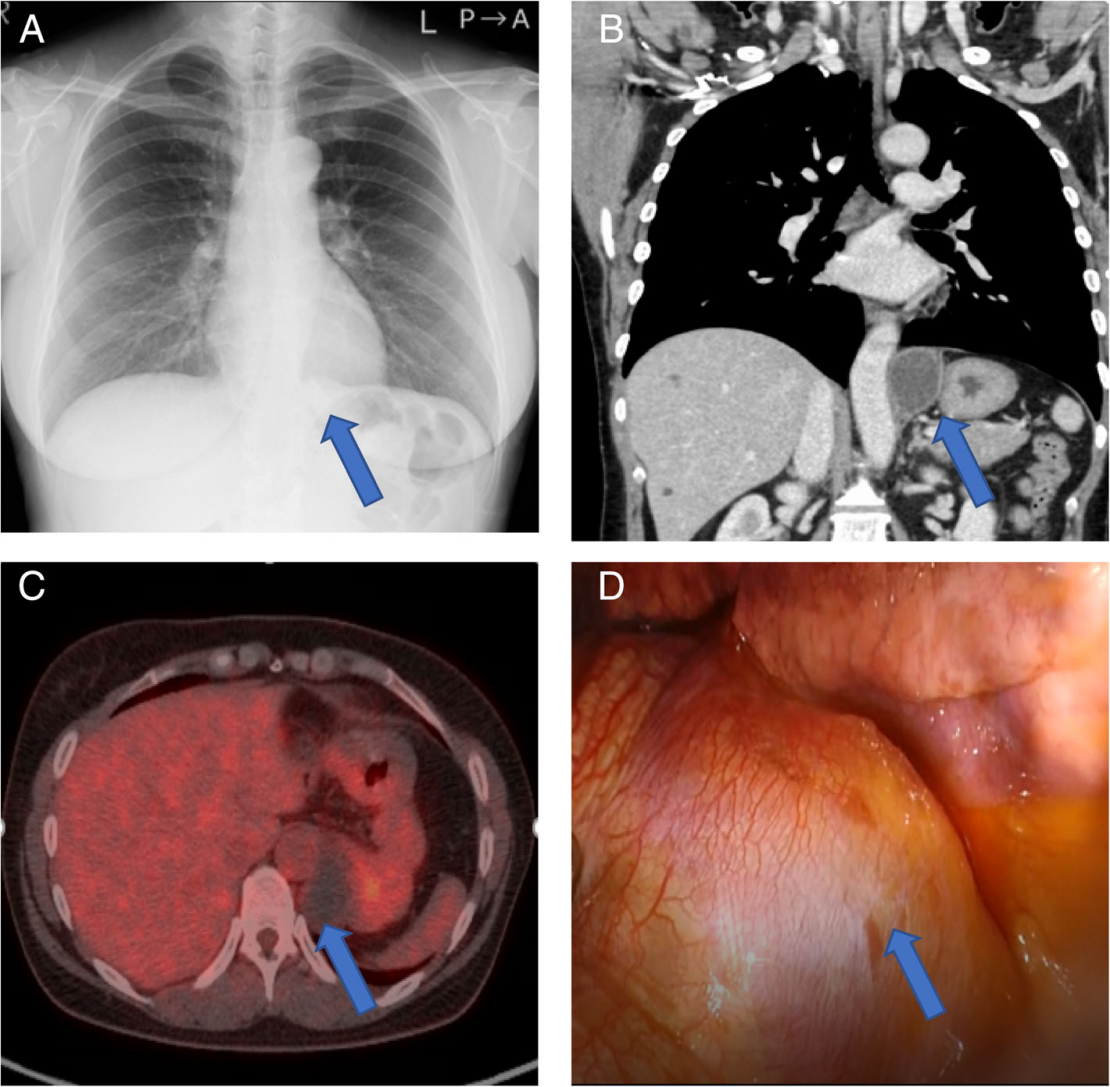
(A) A chest x‐ray showed a nodule near the left diaphragm that overlapped the cardiac shadow. (B) Contrast‐enhanced computed tomography revealed a 5‐cm cystic lesion with a smooth surface and poor contrast effect; however, no clear tumour location could be identified. (C) ^18^F‐fluorodeoxyglucose‐positron emission tomography showed very slight uptake in the tumour. (D) A 5‐cm elevated lesion was found on the dorsal side of the diaphragm
